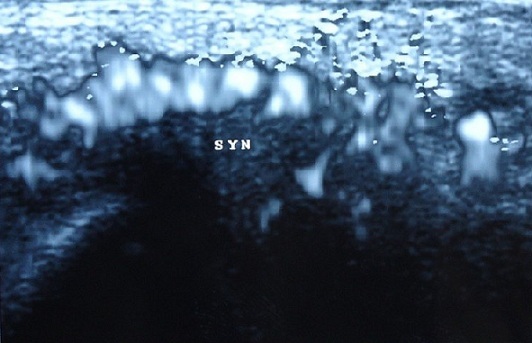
Active synovitis in Doppler with erosion of right sternoclavicular joint
